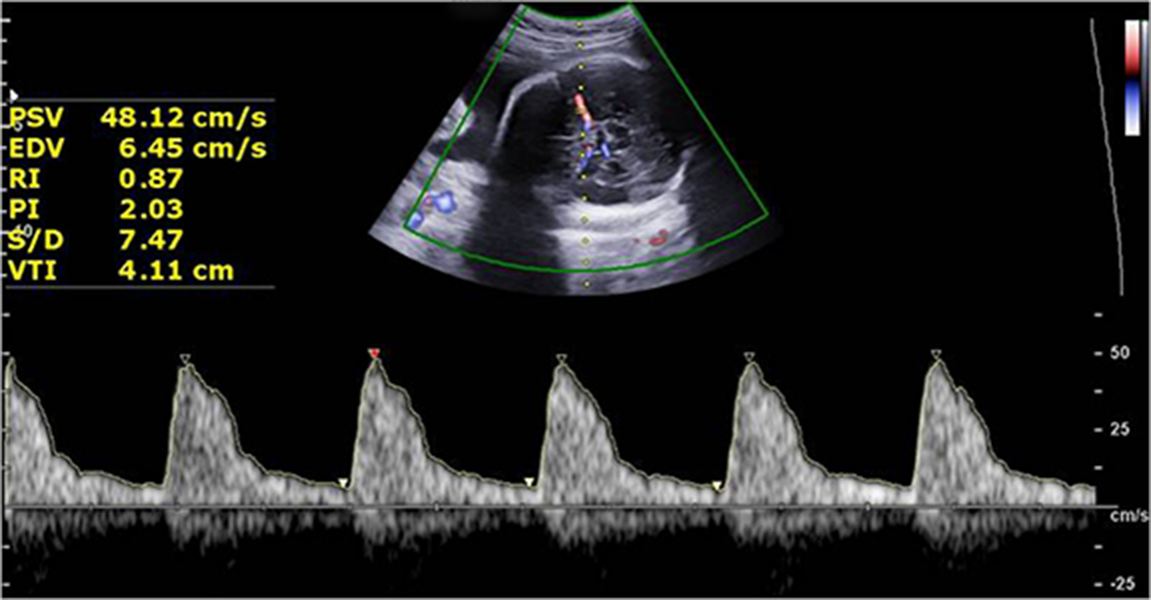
Doppler measurement of the MCA. EDV indicates end‐diastolic velocity; RI, resistive index; S/D, systolic‐to‐diastolic ratio; and VTI, velocity time integral.
